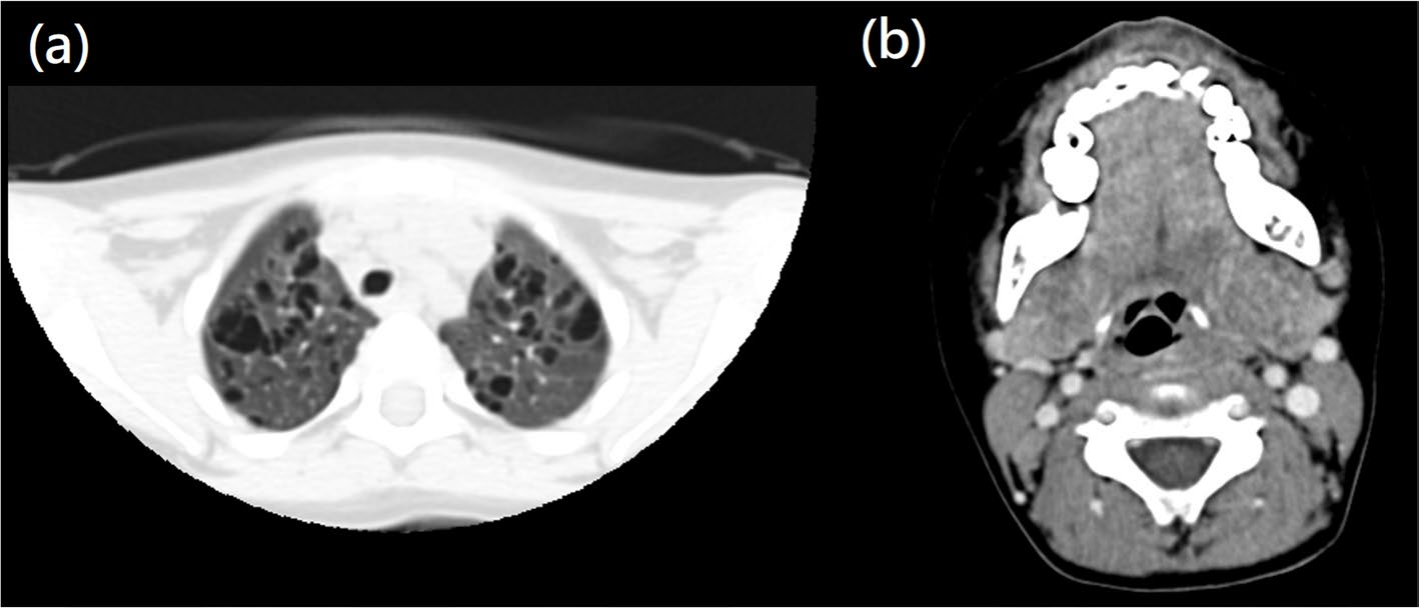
a Axial non-contrast enhanced computed tomography shows the typical pattern of pulmonary Langerhans cell histiocytosis with multiple small confluent thin-walled cysts. b Axial contrast enhanced computed tomography shows enlarged bilateral submandibular glands with multiple non-enhancing hypodense nodules within
